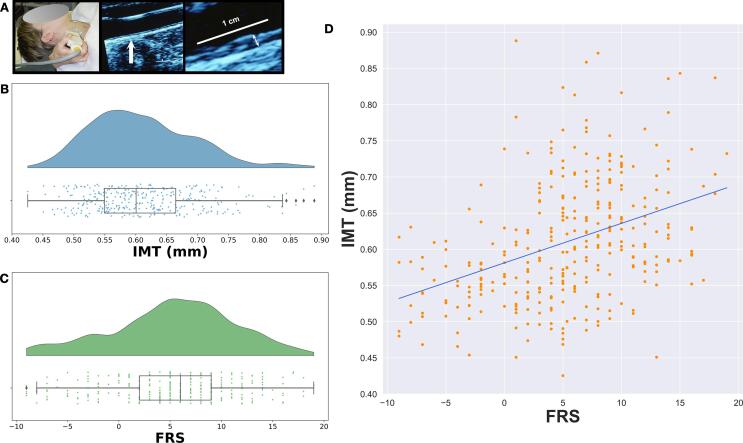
A) Left panel shows CA-IMT acquisition using ultrasound. Middle and right panels show example ultrasound images with the CA-IMT indicated. B) Raincloud plot showing distribution of CA-IMT (mm) in our sample. C) Raincloud plot showing distribution of FRS in our sample. D) Scatterplot showing the linear regression of FRS on CA-IMT. Line of best fit shown in blue. CA-IMT = carotid artery intima-media thickness, FRS = Framingham Risk Score.
